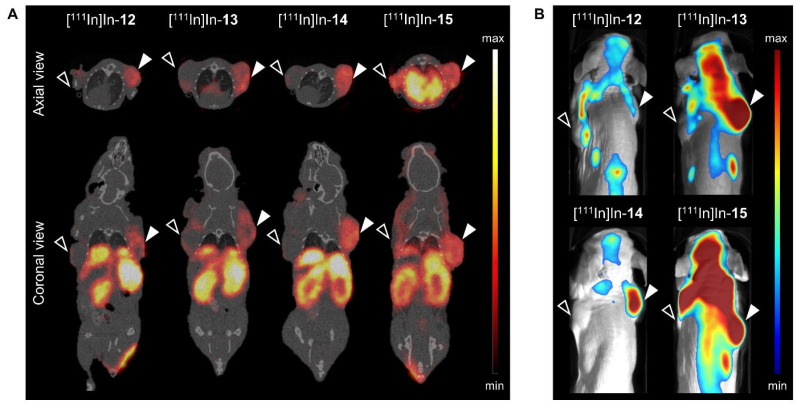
In vivo SPECT/CT (A) and optical (B) images of GRPR-positive PC-3 (
